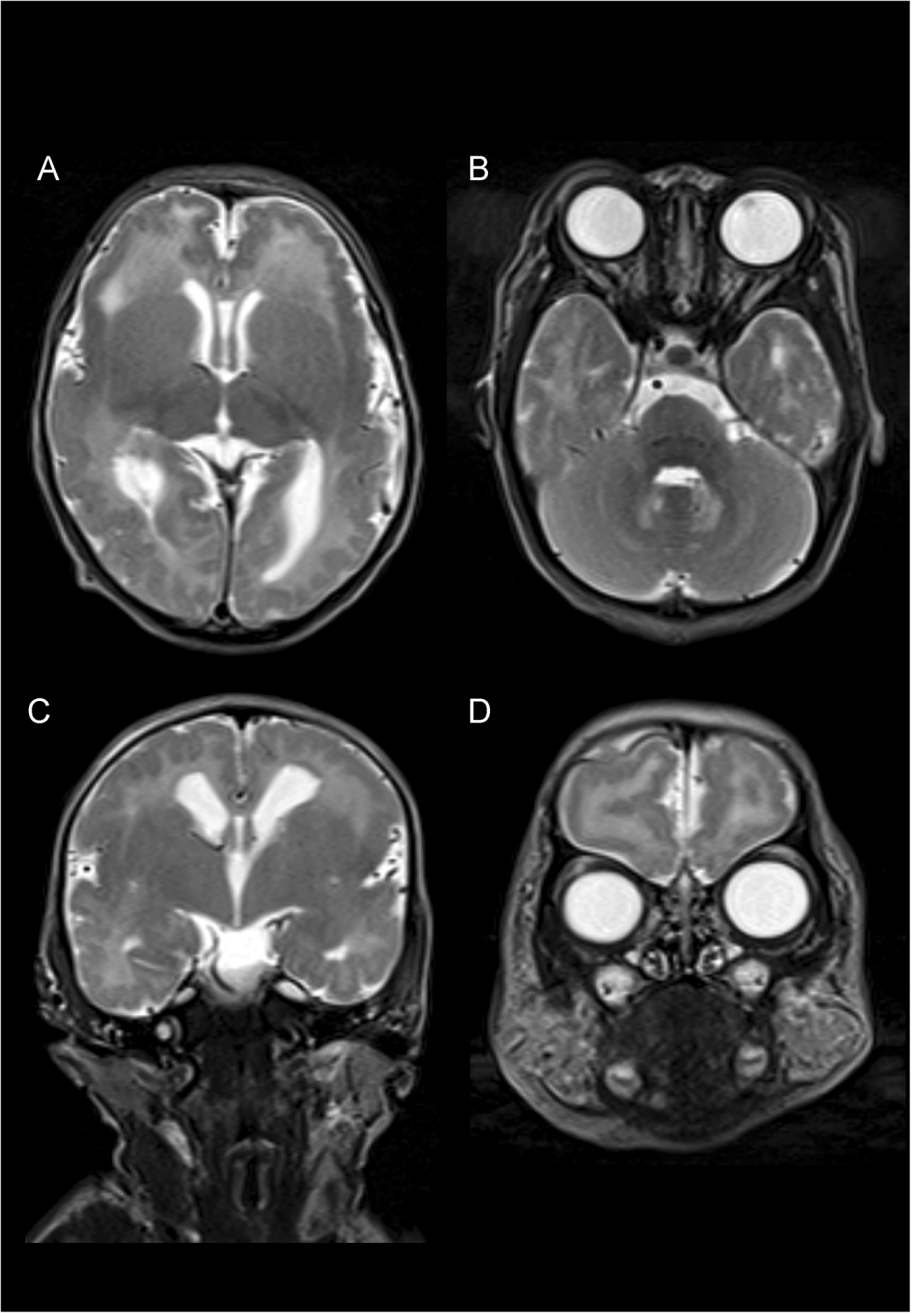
Brain MRI findings. T2-weighted axial (a, b) and coronal (c, d) brain MRI showing bilateral polymicrogyria in the perisylvian and frontal regions.
